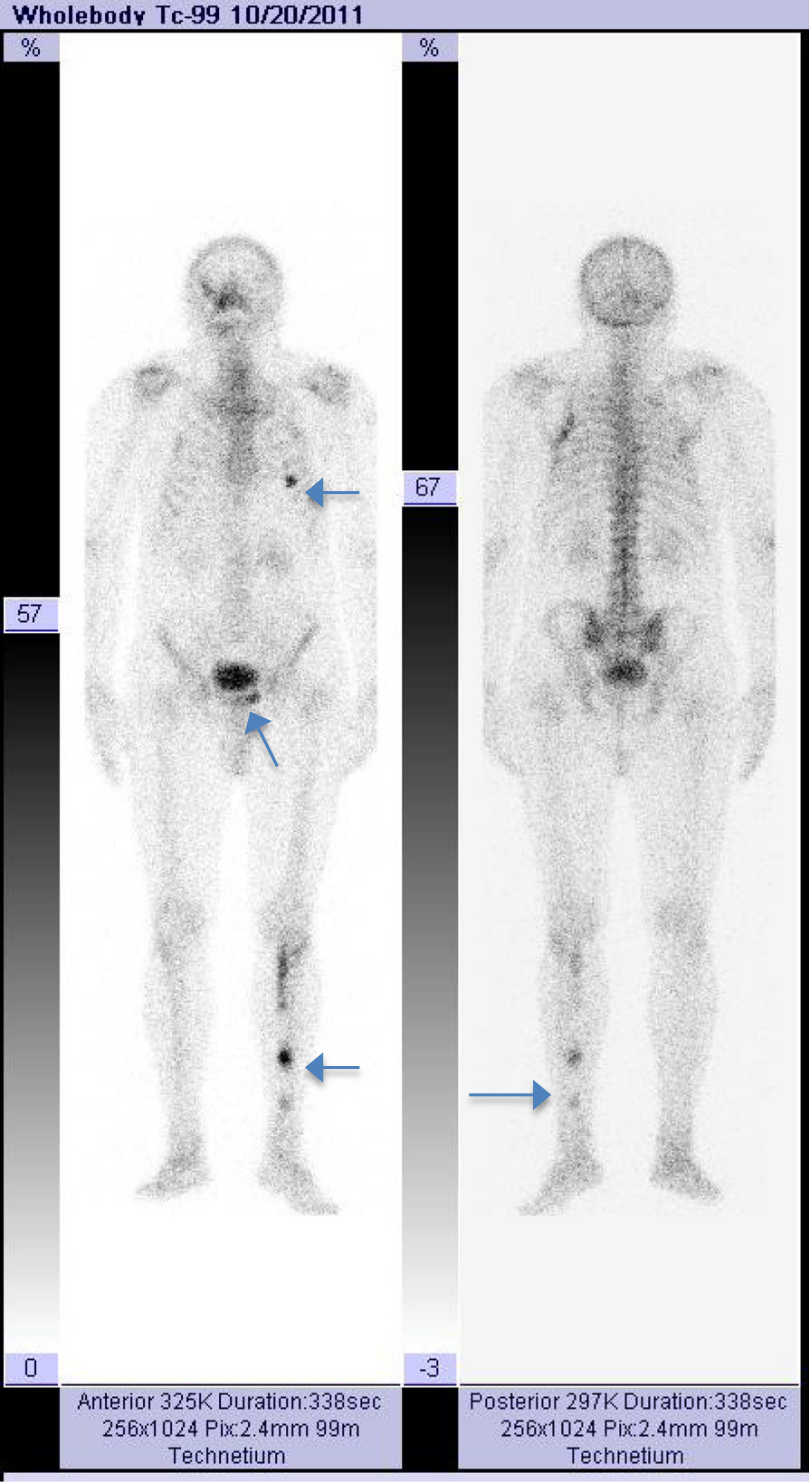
Whole body bone scintigraphy using Tc-99m MDP, showing pathologic uptake of tracer in thorax, pelvic area and left tibia
